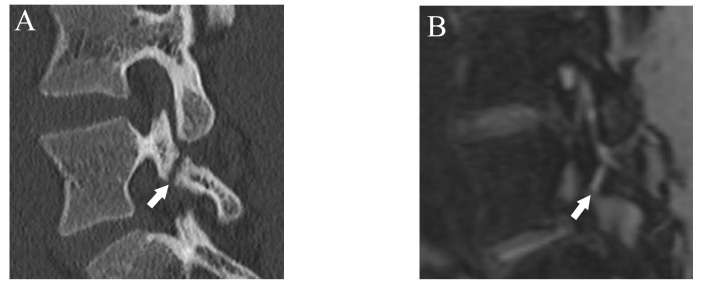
Representative images of grade 3 fracture. The right pars interarticularis of L5 from a 16-year-old male. (A) Sagittal CT image showing a complete fracture across the whole pars interarticularis (arrow). (B) Sagittal DESS image showing a linear high signal corresponding to the complete fracture (arrow).
